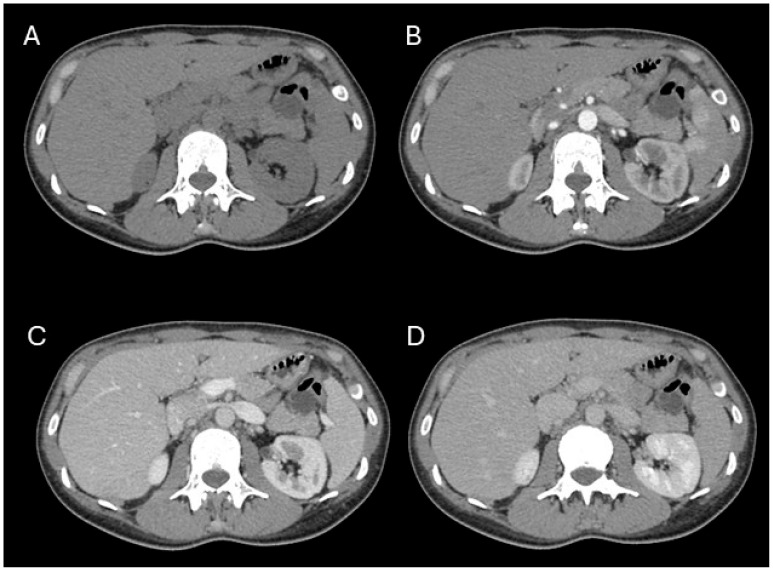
Axial contrast-enhanced CT images of the abdomen demonstrating a well-defined, lobulated cystic lesion in the pancreatic tail, consistent with a cystic pancreatic hamartoma. The lesion is visible across multiple phases without evidence of solid enhancing components. (A) Pre-contrast phase. (B) Arterial phase. (C) Portal venous phase. (D) Delayed phase. The lesion remains hypoattenuating relative to the enhancing pancreatic parenchyma throughout all phases, with no communication with the main pancreatic duct or surrounding infiltration.
